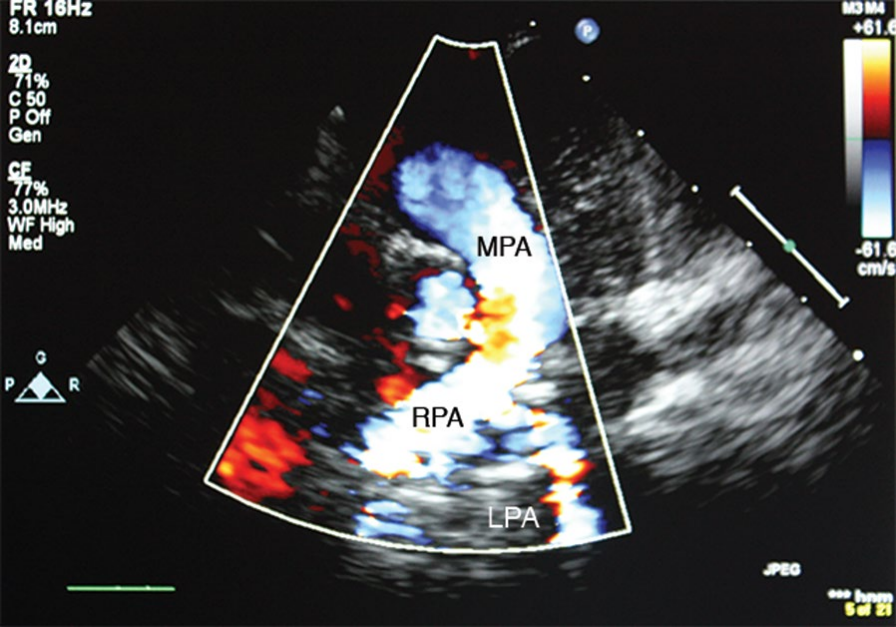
Transthoracic echocardiogram with Doppler showing main pulmonary artery (*MPA*), right pulmonary artery (*RPA*) and left pulmonary artery (*LPA*) flow. Note turbulence within the LPA segment as it courses between the trachea and esophagus
